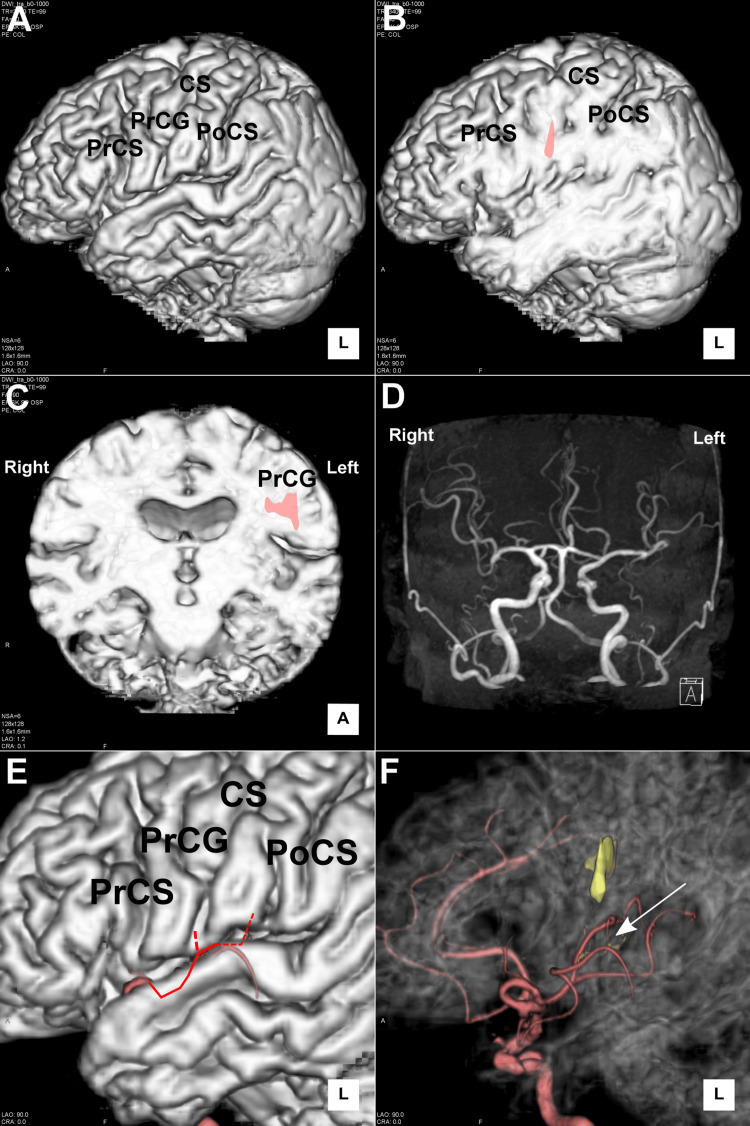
Lesion and middle cerebral artery overlaid on the MRI surface (after thrombolysis). Infarct overlaid on the MRI surface rendering (A, B). Sagittal section at the infarction (B). Coronal section of the MRI surface rendering (C). The pink lesion represents the infarction in the precentral gyrus. Magnetic resonance angiography (MRA) (D). MRA overlaid on the MRI surface rendering (E), transparent image (F). The red line indicates the superior trunk of the middle cerebral artery from which the central artery originates. The red dotted line indicates a double cortical artery of the central artery. Note the configuration of the opercular portion of the middle cerebral artery, which is thought to be the central artery (shown in a red dotted line). CS: Central sulcus; PrCG: precentral gyrus; PrCS: precentral sulcus; PoCS: postcentral sulcus.
